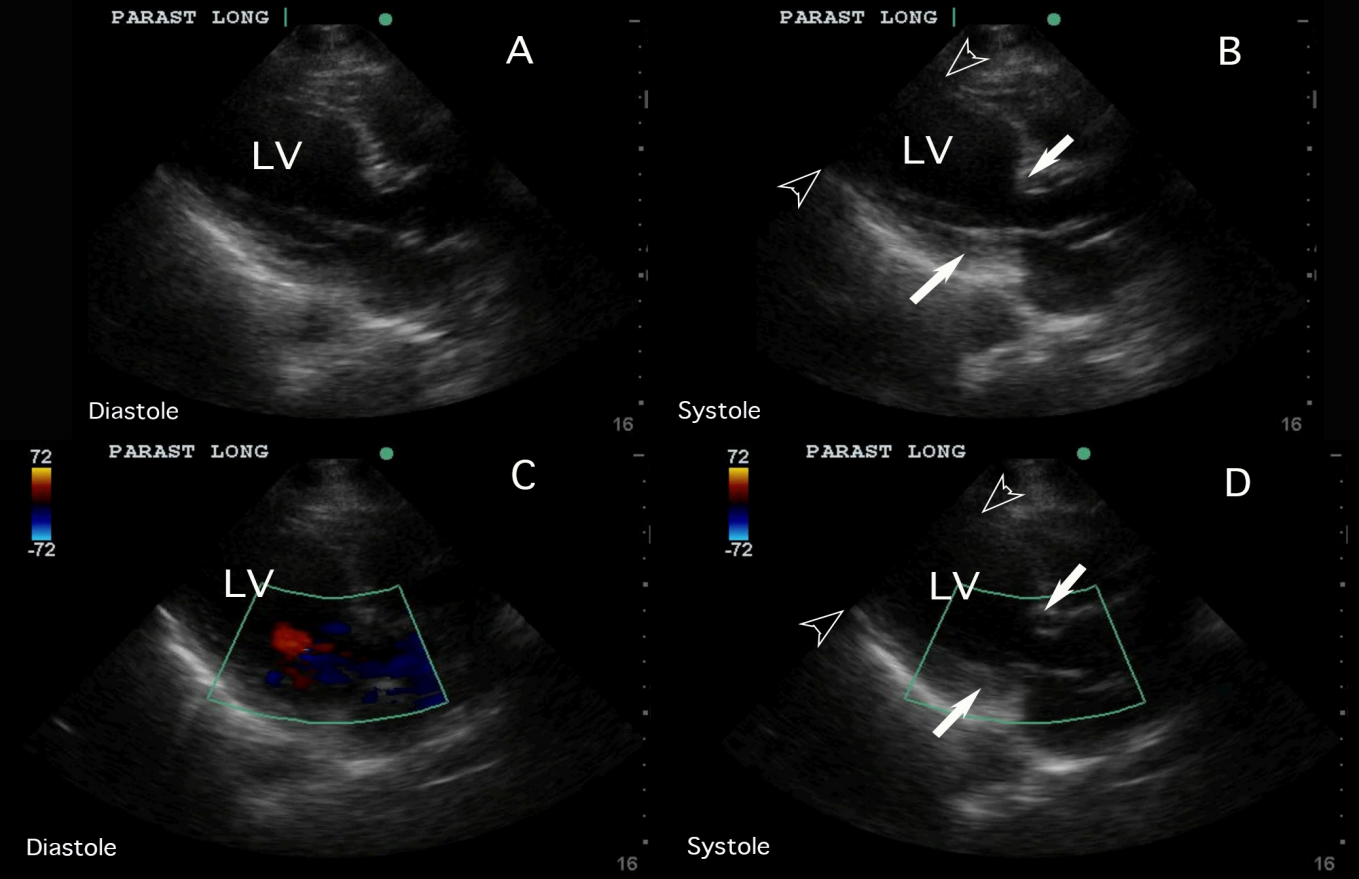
These parasternal long-axis views, both with (panels C and D) and without (panels A and B) color, demonstrate the systolic apical ballooning of the left ventricle (LV) with preserved contraction of the basal segments. The apical portions of the interventricular septum and free wall of the left ventricle do not show significant movement when systole (panels B and D) is compared to diastole (panels A and C), indicating impaired contraction of the apical segment (outlined arrowheads). The left ventricular basal segments, however, move closer together in systole (panels B and D) to show preserved contraction of this portion of the heart (solid arrows).
